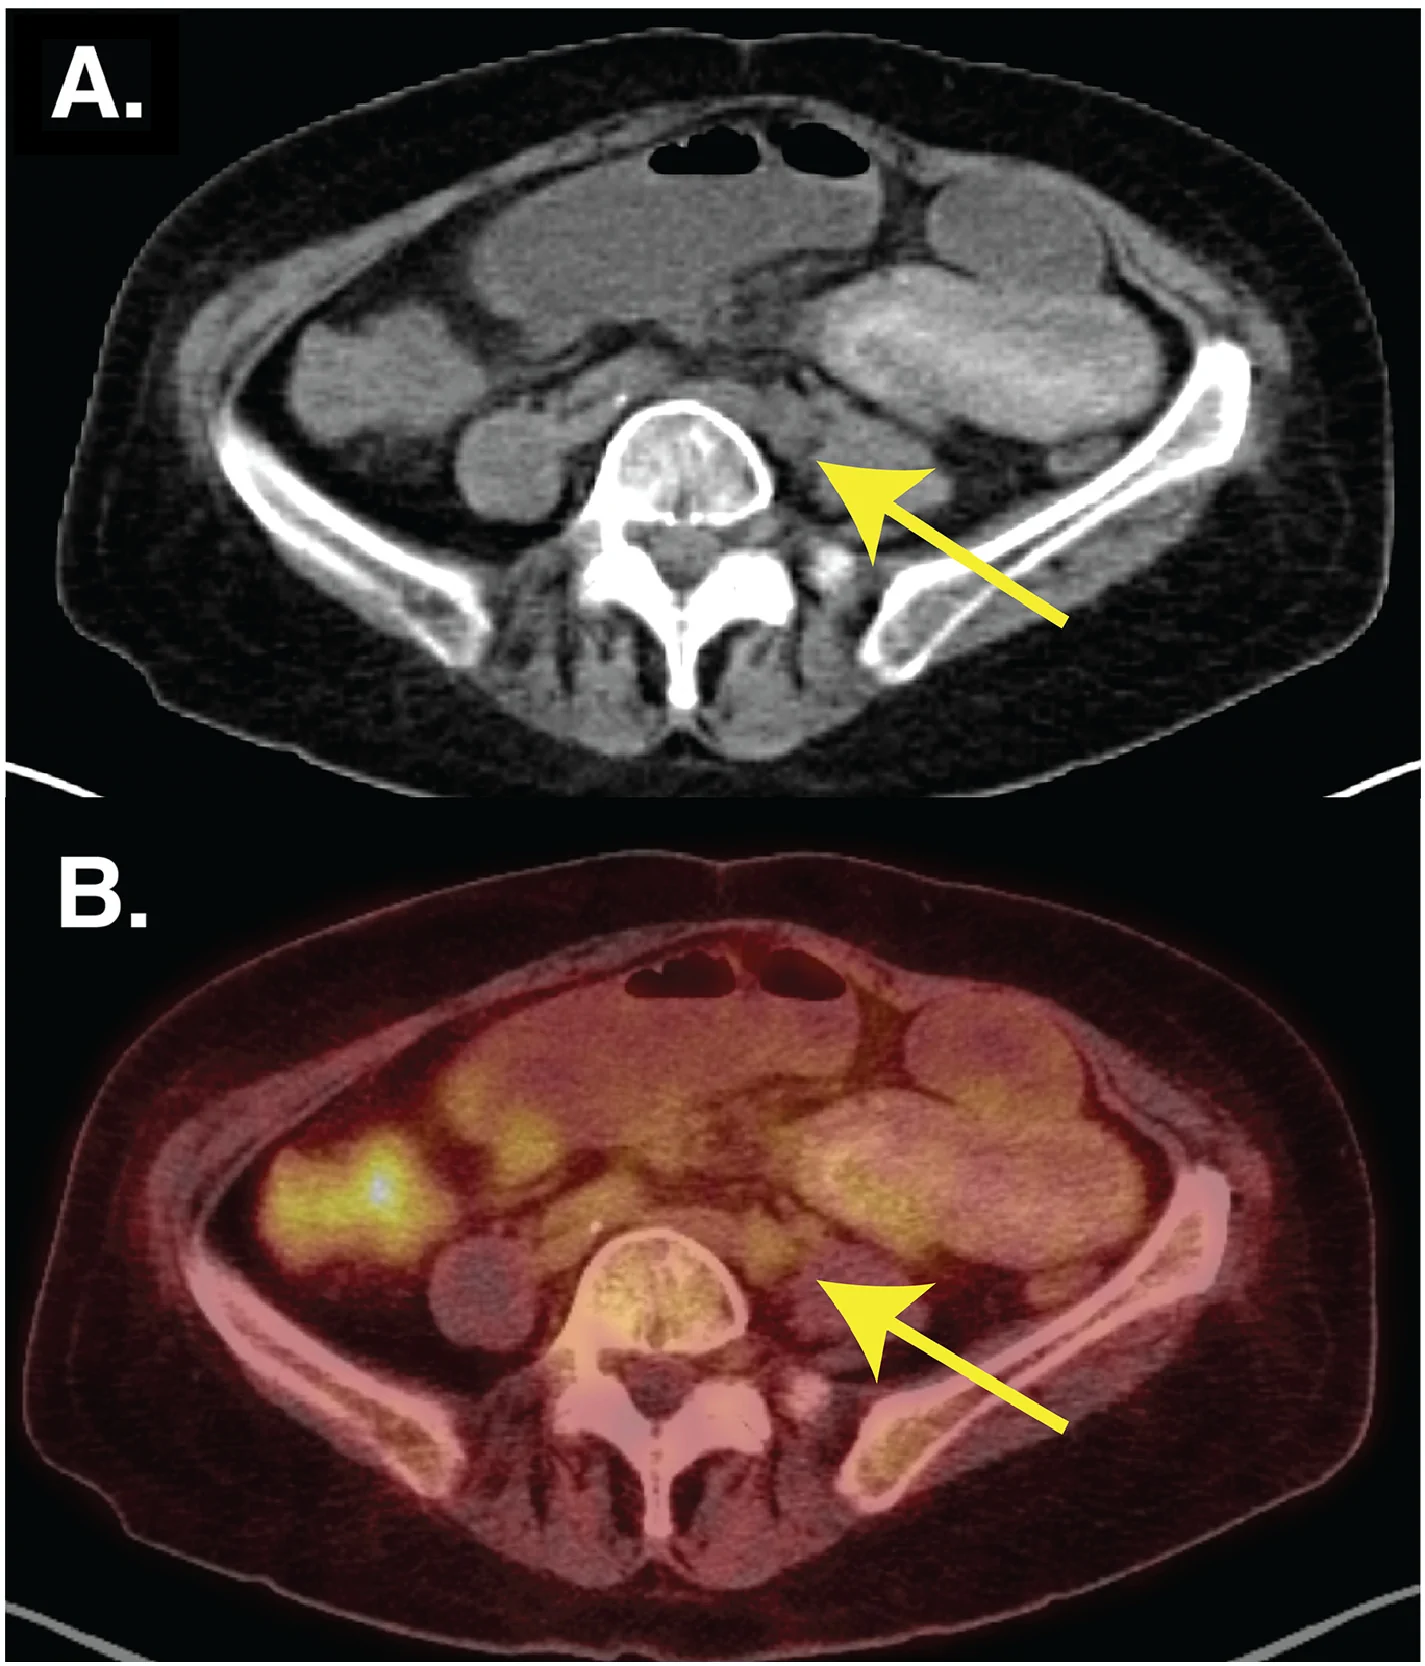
Resolution of biopsied DOTATATE-avid lymph node. CT (A) and fused PET/CT (B) show resolution of the DOTATATE-avid left common iliac lymph node (yellow arrow).
